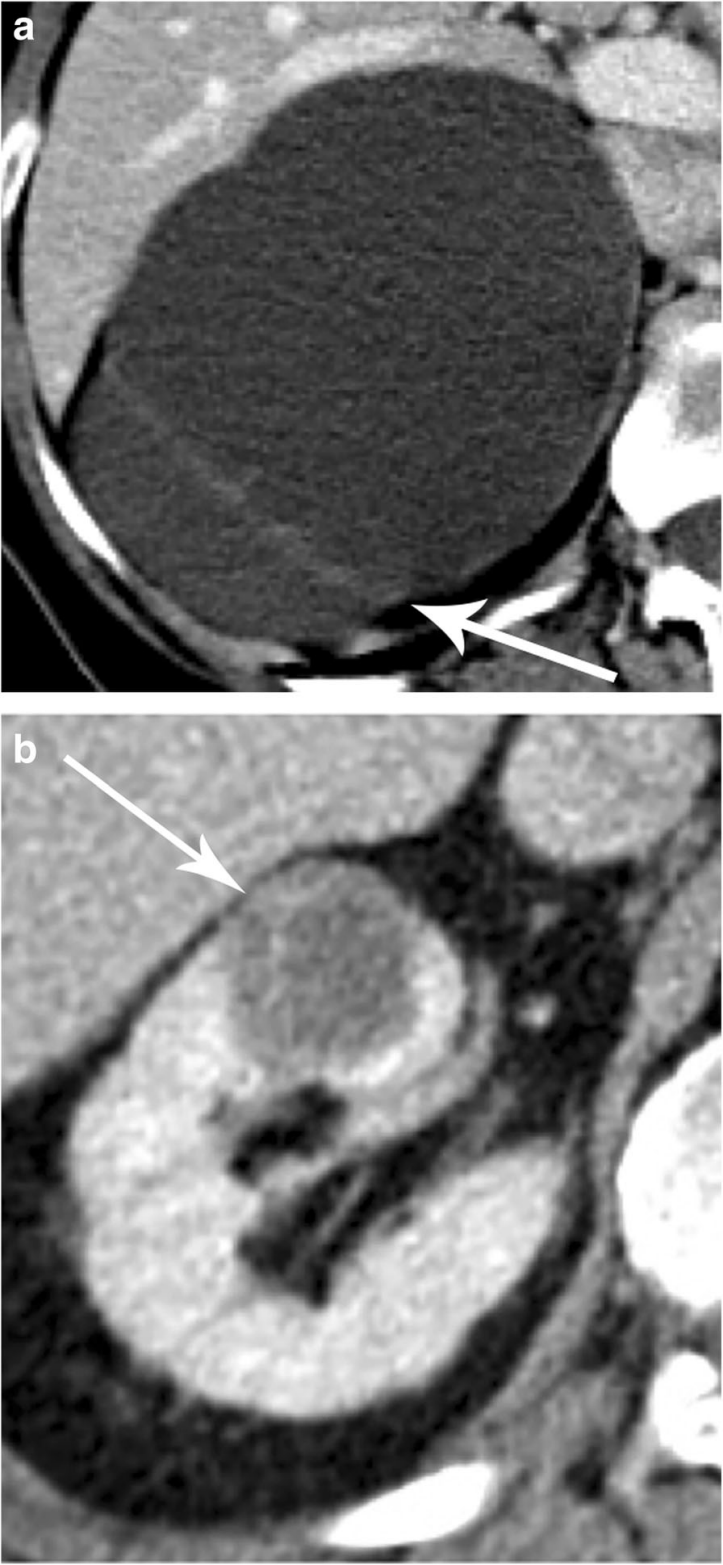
Bosniak IIF, differentiation between low and more complex lesions. a Axial contrast-enhanced CT depicting a large cyst with a solitary thick septum. The cyst should be followed because the septum is thick, but suspicion of malignancy is very low (arrow). b Axial contrast-enhanced CT demonstrating a cyst with multiple septa. This complex appearance does not completely rule out a possible cystic tumor (arrow)
